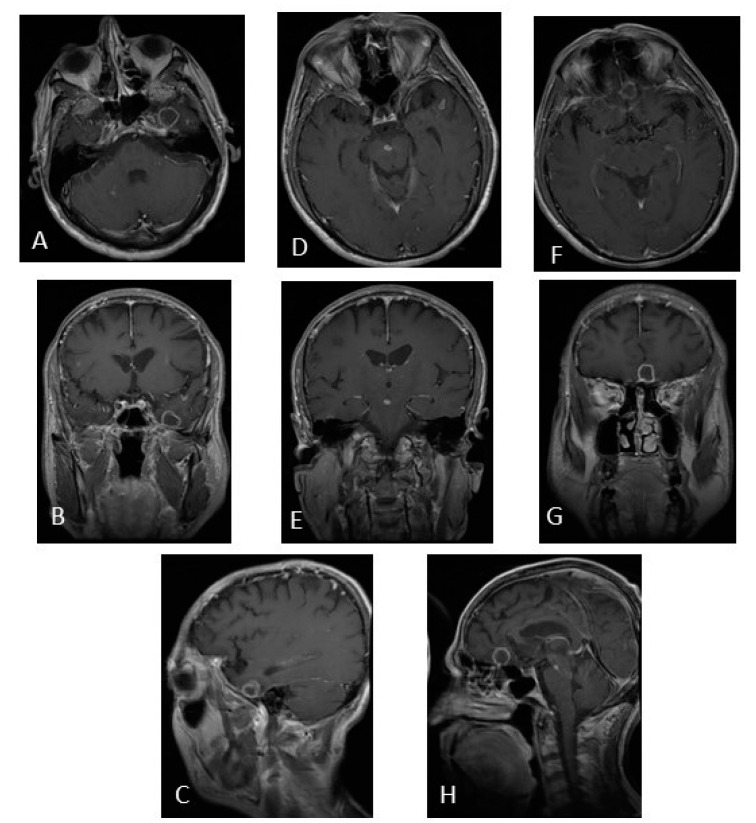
MRI of brain post-contrast T1-weighted image. (A–C) Tuberculoma in the left temporal lobe. (D,E) Tuberculoma in the midbrain. (F–H) Tuberculoma in the frontal lobe. Sixty-four-year-old patient, with previous use of infliximab, with a fever of 6 months of evolution. Clinical improvement at 2 weeks, and imaging improvement at 9 months after starting antituberculous drugs. (The photo is from our image gallery).
